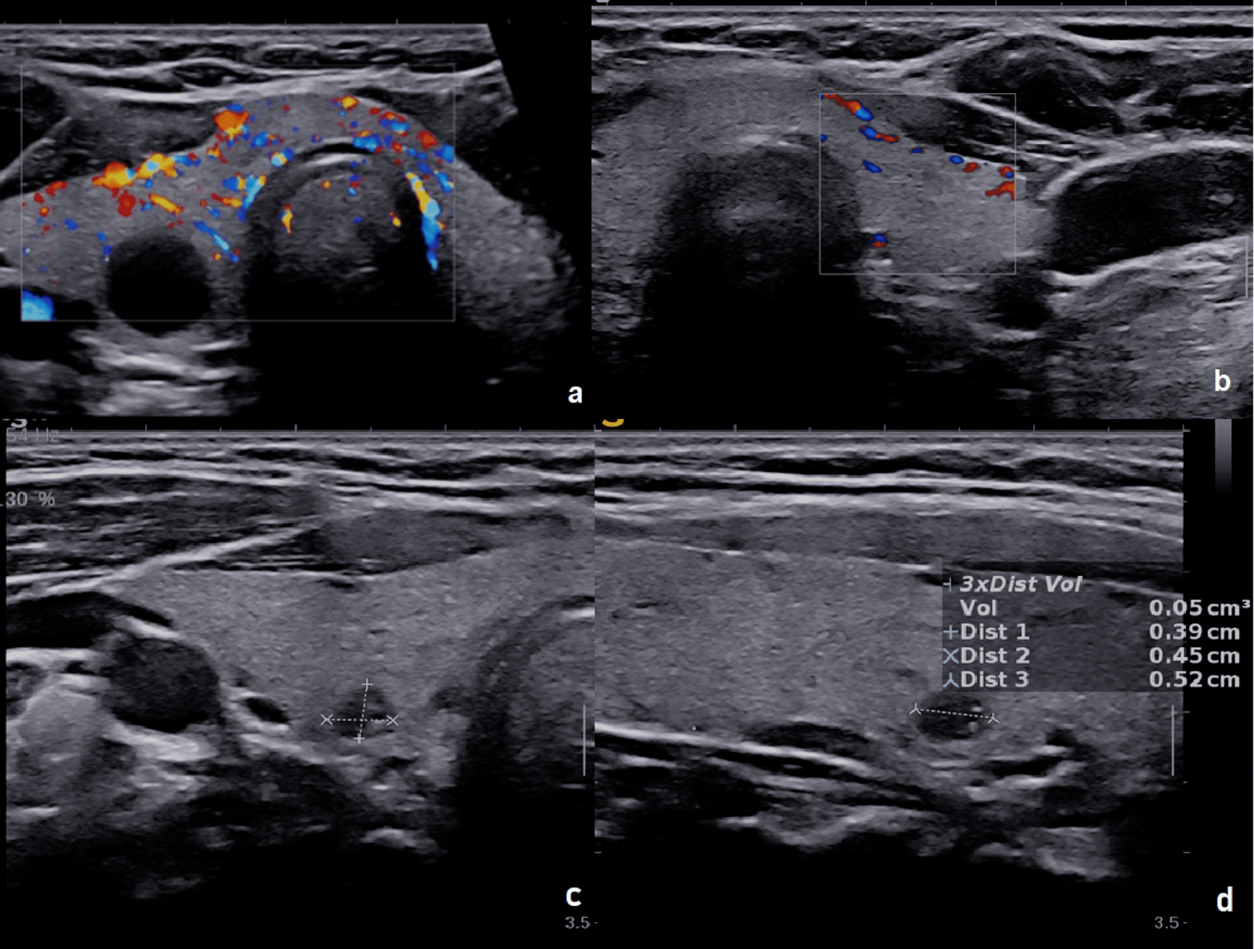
Thyroid ultrasounds. (a) Axial thyroid ultrasound showing diffuse vascular hyperemia, consistent with acute thyroiditis, and a benign cystic lesion with fine non-vascularized septations (14 × 13 × 11 mm) in the inferior pole of the right thyroid lobe (EU-TIRADS 3). (b) Axial thyroid ultrasound with color Doppler performed six weeks later showing normal vascularization. (c, d). Axial ultrasound with color Doppler performed six weeks later showing regression of the cyst (3.9 × 4.5 × 2.5 mm) and reclassification as EU-TIRADS 2.
